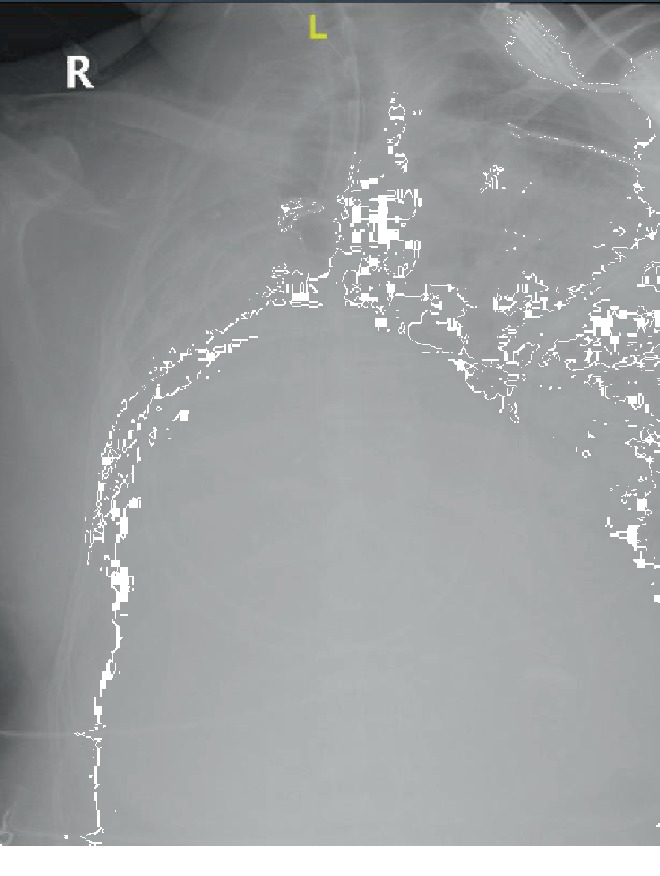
Chest X-ray showing significant worsening of the L-sided infiltrate, leading to complete opacification of the left lung.
